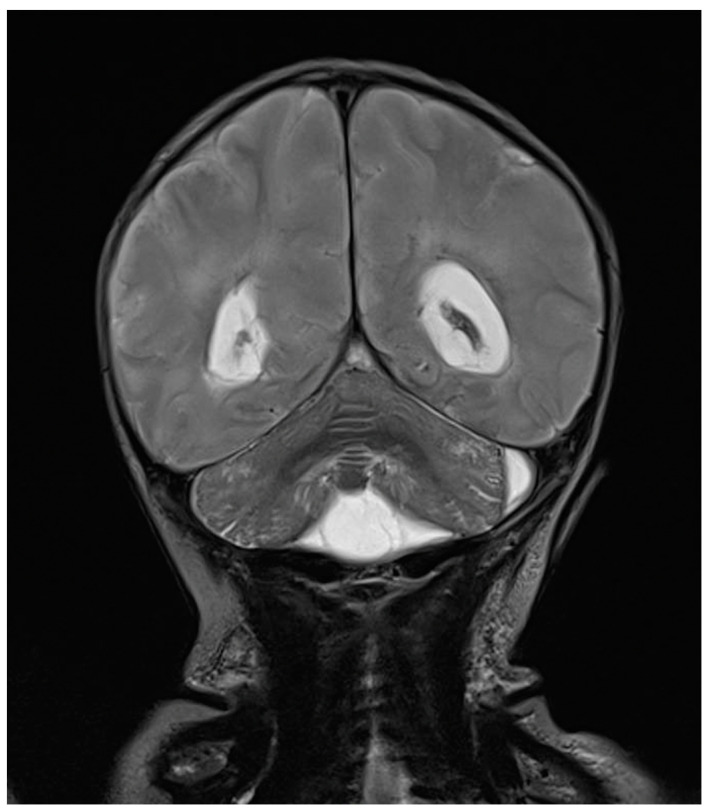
Brain MRI in T2 sequence: picture compatible with meningoencephalitis associated with hypoxic suffering. Infratentorial empyema with small amounts of pus in ventricles supratentorially. Enlargement of lateral ventricles.
